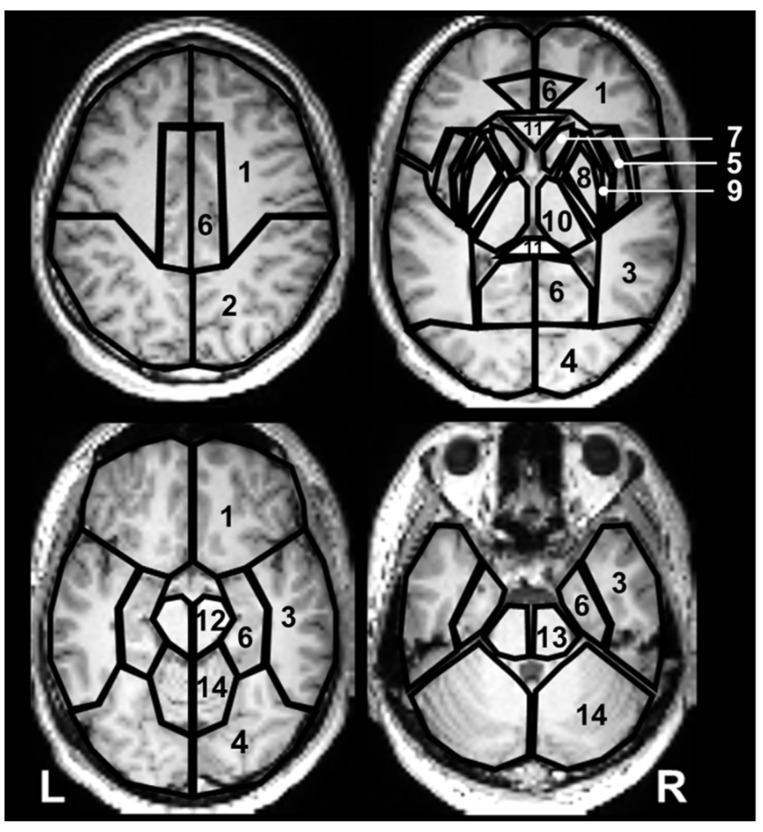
The outline of the regions of interest (ROIs) overlaid on the axial sections of normalized T1-weighted 3D magnetization-prepared rapid acquisition gradient echo (MPRAGE) images of a participant. Each ROI is placed on the either side of the frontal lobe (1); parietal lobe (2); temporal lobe (3); occipital lobe (4); insular lobe (5); limbic lobe (6); caudate (7) and lentiform nuclei (8); claustrum (9); thalamus (10); sublobar white matter (11) containing corpus callosum, external and internal capsules, and periventricular white matter; midbrain (12); pons (13); and cerebellar hemispheres (14).
